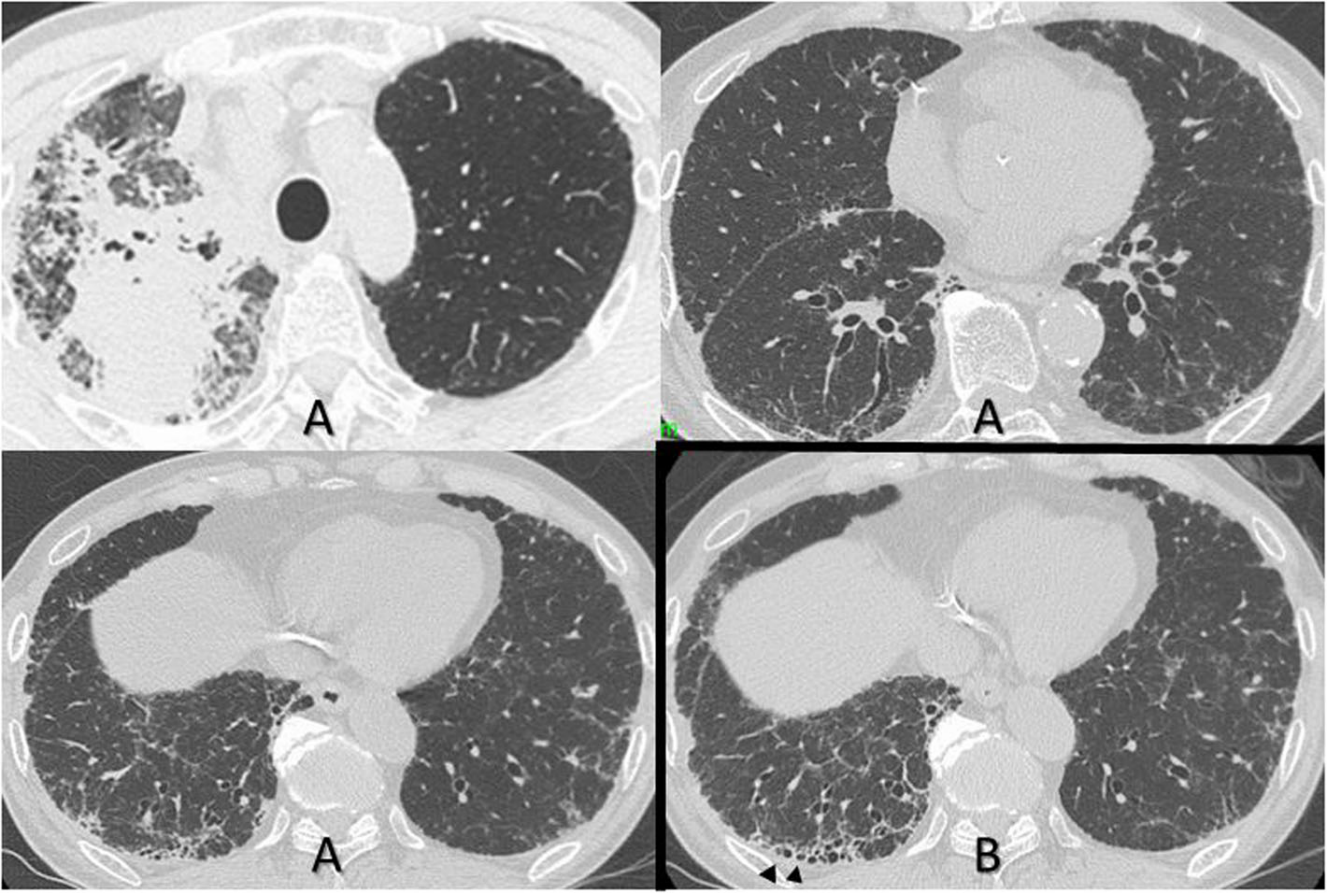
(a) Chest high-resolution computed tomography performed at initial presentation showed a mass lesion in the right upper lobe as the primary lung cancer and interstitial abnormality predominantly in the lower lobe. The interstitial abnormality was basal predomoinant and showed reticulation with peripheral traction bronchiectasis and bronchioloectasis, which was probably compatible with usual interstitial pneumonia pattern. (b) One year and 3 months after initial presenteation, honeycomb lesions appeared in the lower lobe (arrowheads)
